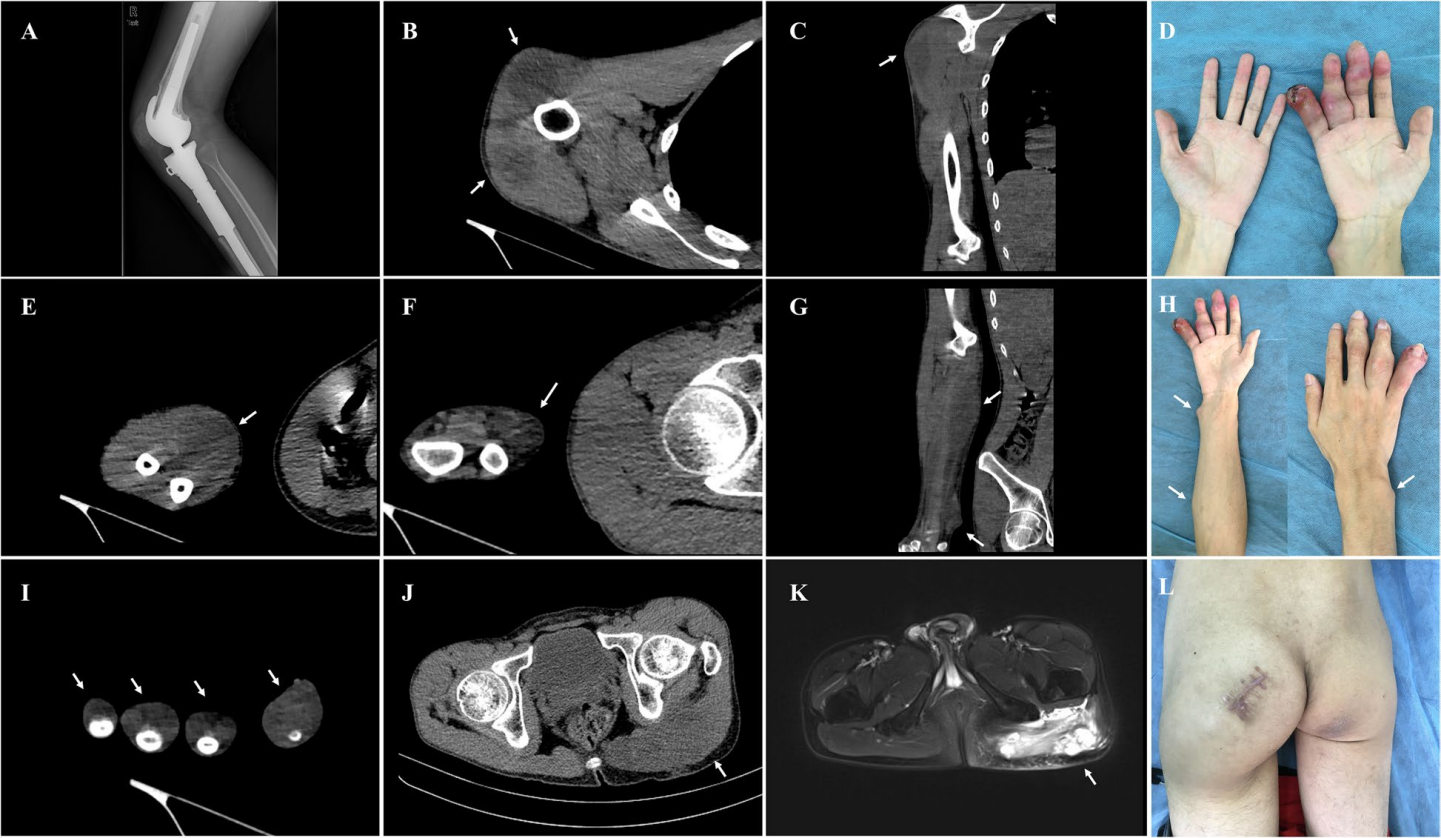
The radiological findings of the metastatic lesion from osteosarcoma. The radiograph showed no local recurrence around the right knee (A). The CT scan of whole body demonstrated multiple soft masses without bone destruction in the right deltoid muscle (B, C), right forearm (E, F, G, H), the 2, 3, 4, 5 digits of the right hand (D, I) and left gluteal maximus muscle (J, L). Corresponding axial fat-saturated T2-weighted MRI image of the pelvis (K) depicted a soft tissue mass with high signal intensity in the gluteal maximus muscle
